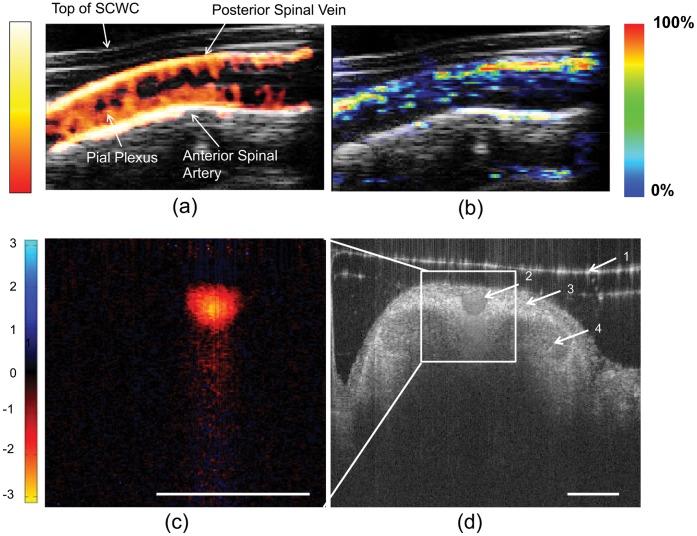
SCWC permits structural, functional and oxygenation imaging of the intact spinal cord vasculature *in situ*. (A) Power Doppler ultrasound (color) overlaid on a B-mode structural ultrasound (gray-scale) image obtained through the polycarbonate SCWC along a longitudinal section of the normal spinal cord *in vivo* (device is shown in Figure 1F). The power Doppler depicts vascular architecture in several vessels of the spinal cord. The color bar represents the signal intensity. (B) Corresponding multispectral photoacoustic imaging of the same cross section of normal spinal cord permitted *in situ* measurement of hemoglobin oxygen saturation in the anterior spinal artery and posterior spinal vein. It demonstrated that the cord is well oxygenated. The color bar represents the relative hemoglobin oxygen saturation level. (C) Cross-sectional Doppler OCT image demonstrated significant blood flow in the posterior spinal vein. The color bar represents the phase-shift of the backscattered light in radians which is proportional to the velocity of the red blood cells in the axial direction. (D) Corresponding structural OCT image of the spinal cord permitted visualization of key spinal cord features, including the glass coverslip (1), anterior spinal vein (2), white matter (3), and grey matter (4) of the intact cord. Scale Bars = 500 µm (A–D). SCWC = spinal cord window chamber. OCT = optical coherence tomography.
